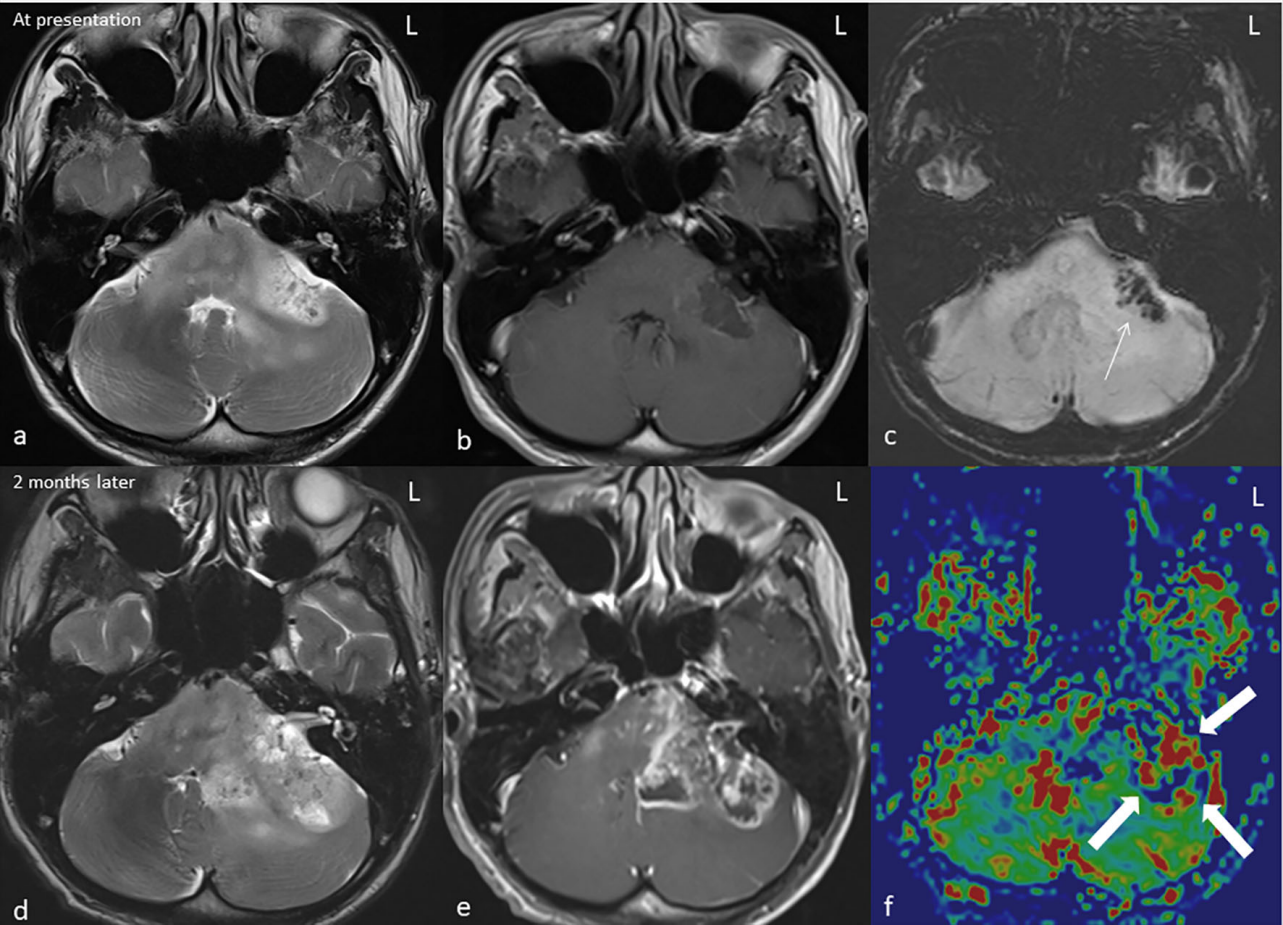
MRI* of the brain in axial T2W (A, D), T1W* post gadolinium (B, E), SWI (C), and rCBV* colour map (F) at presentation (top row) and at 2 months (bottom row). The left middle cerebellar peduncle mass demonstrates a heterogeneous hyperintense signal on T2 with minimal peripheral enhancement on T1 post-contrast. The hypointensity on SWI* indicates presence of hemosiderin (arrow). The lesion significantly increases in size on the follow-up MRI (C, D) causing significant mass effect; the enhancement is more heterogeneous with irregular rim enhancement and central necrosis. Increase in rCBV at the enhancing region of the rCBV colour map indicates a high-grade tumour (block arrow). *Magnetic resonance imaging, T2-weighted, *T2-weighted, susceptibility-weighted imaging, and *relative cerebral blood volume.
